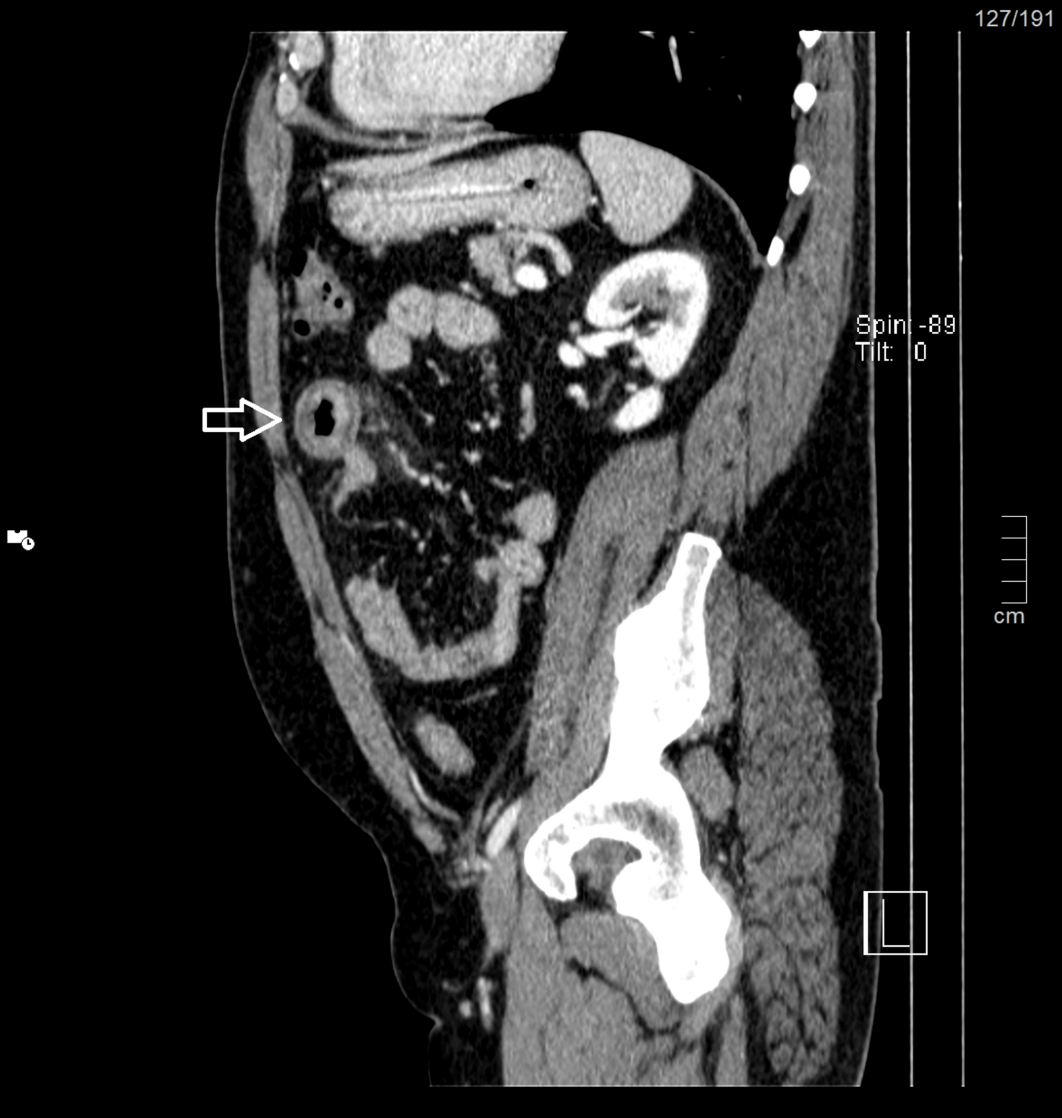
Sagittal plane of abdominal CT The inflammatory process of the jejunal mucosa with densification of the adjacent peritoneal fat and mesenteric vascular engorgement is observed (arrow).
